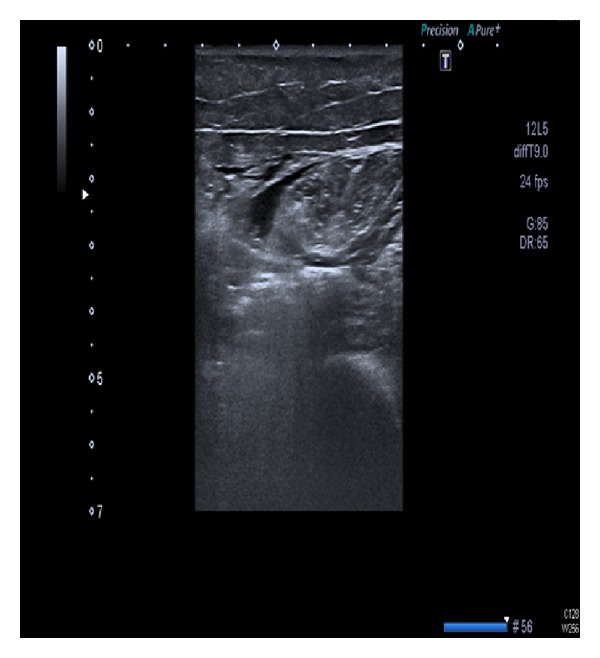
Ultrasound of thigh: edema and fluid bands around anterior thigh muscles.
